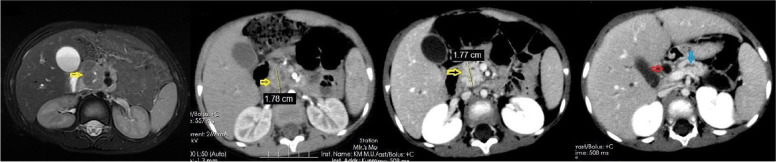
Magnetic resonance imaging (a) (in local children’s hospital, but not reported at that time) and computed tomography scan (b) (in our hospital) show a tumor with an inhomogeneous density at the pancreatic head (yellow arrow). In the delayed phase, the mass shows obvious enhancement (c) with the dilated common bile duct (red arrow) and pancreatic duct (blue arrow, d)
